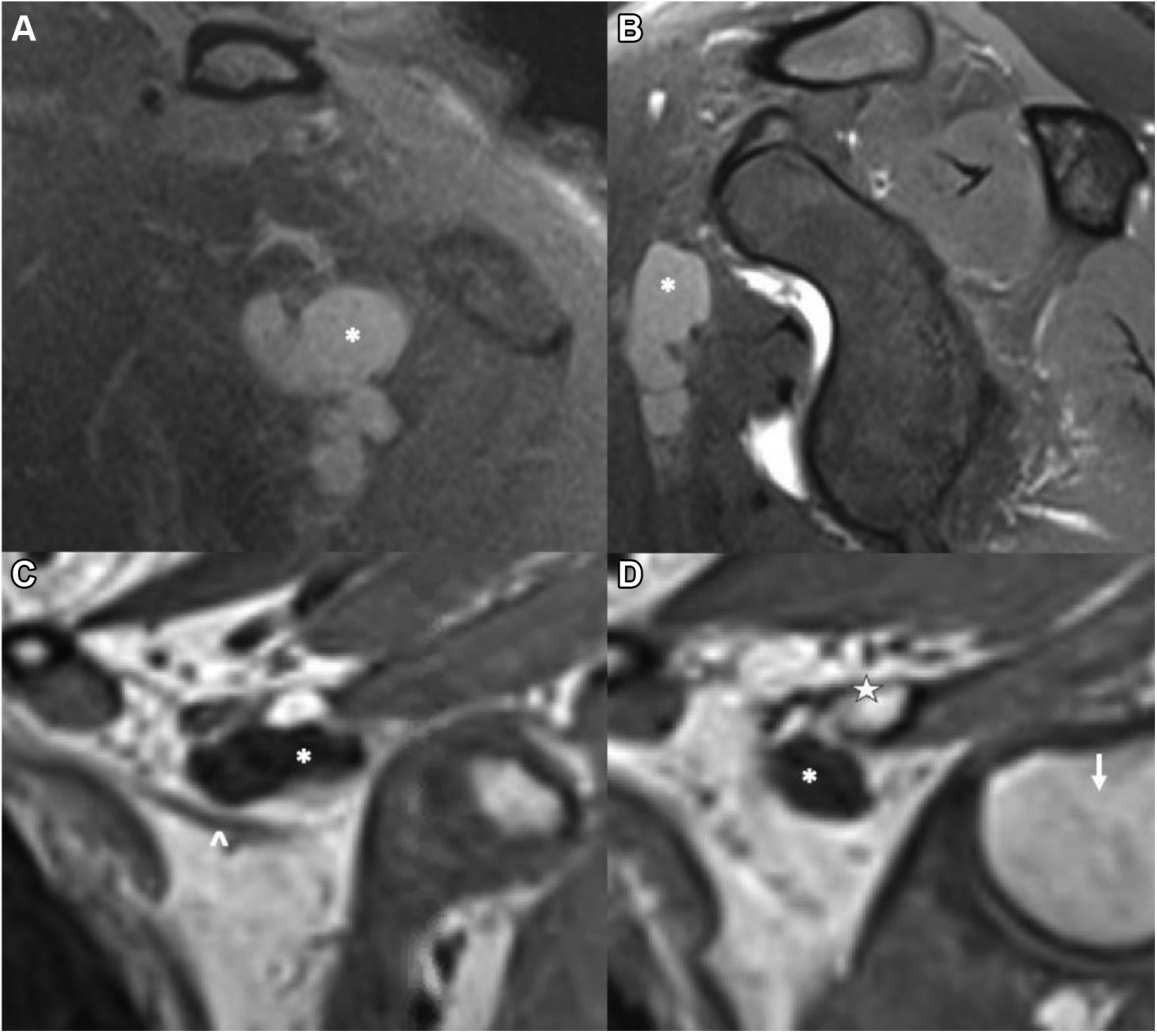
Large subcoracoid cyst noted on MRI of the left shoulder in a patient with vague, deep pain about the anterior shoulder and upper chest, worse with repetitive activity. (A) Coronal view, cyst marked with white ∗. (B) Sagittal view, cyst marked with white ∗. Dedicated MRI of the brachial plexus was performed, showing proximity of the subcoracoid cyst to the brachial plexus. (C) Anterior coronal slice, cyst marked with white ∗, plexus marked with white ˆ. (D) Posterior coronal slice, cyst marked with white ∗, coracoid marked with white start, humeral head marked with *white downward arrow*. The patient was treated with arthroscopic pectoralis minor release, cyst decompression, and brachial plexus neurolysis, with resolution of her symptoms and return to activity. *MRI*, magnetic resonance imaging.
